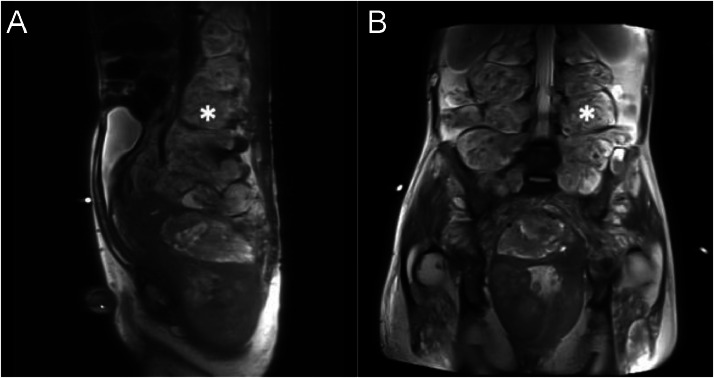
(A) Sagittal and (B) coronal projection of T2-weighted MRI showed the multilevel lobulated mass with a hyperintense signal with the target sign on T2WI extending along right and left lumbar neural foramina (asterisk).
